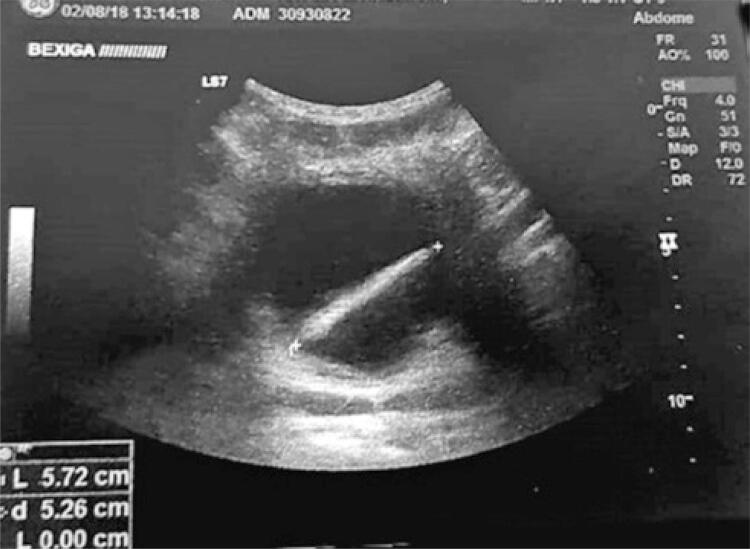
Bladder ultrasound demonstrating a 4cm linear hyperechoic image
